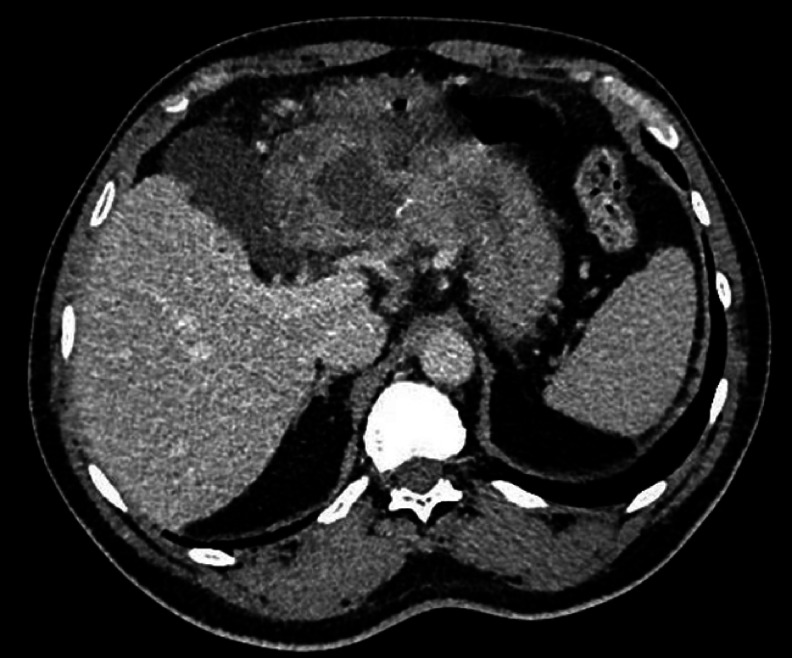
Initial CT scan showing an antral perigastric collection measuring 4 cm in diameter and containing a hyperdense linear image suggestive of a bone or fishbone foreign body.
